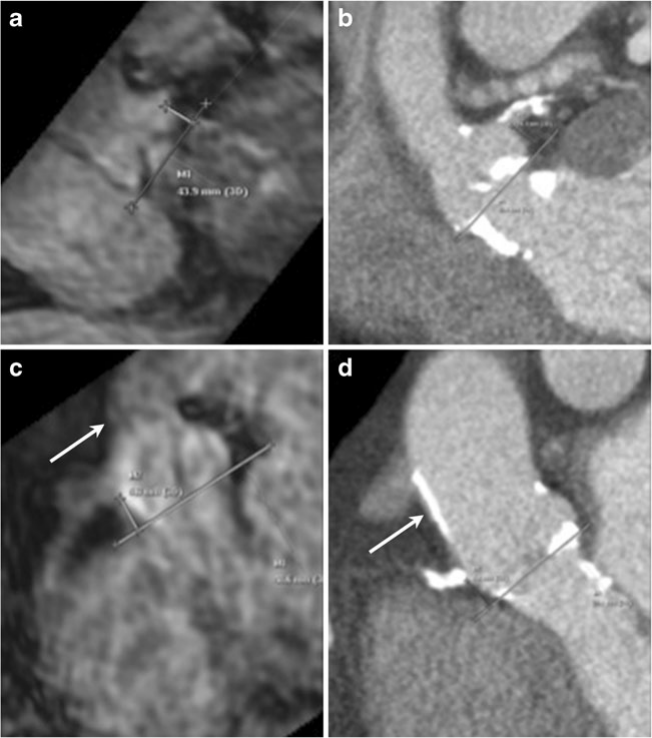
Assessment of coronary ostial height on MRI. Navigator-assisted 3-D SSFP stack of the aortic root in the late diastolic phase is acquired. Ostial height is measured from the aortic valve annular plane (a: left coronary artery, c right coronary artery). In the same patient corresponding CT images (b: left coronary artery, d right coronary artery). This MRI sequence can be also be used to assess sinus of valsalva height and width. Note the dark appearance of the anterior aortic wall and the left ventricle outflow tract due to extensive calcifications, easily seen on the corresponding CTA images
